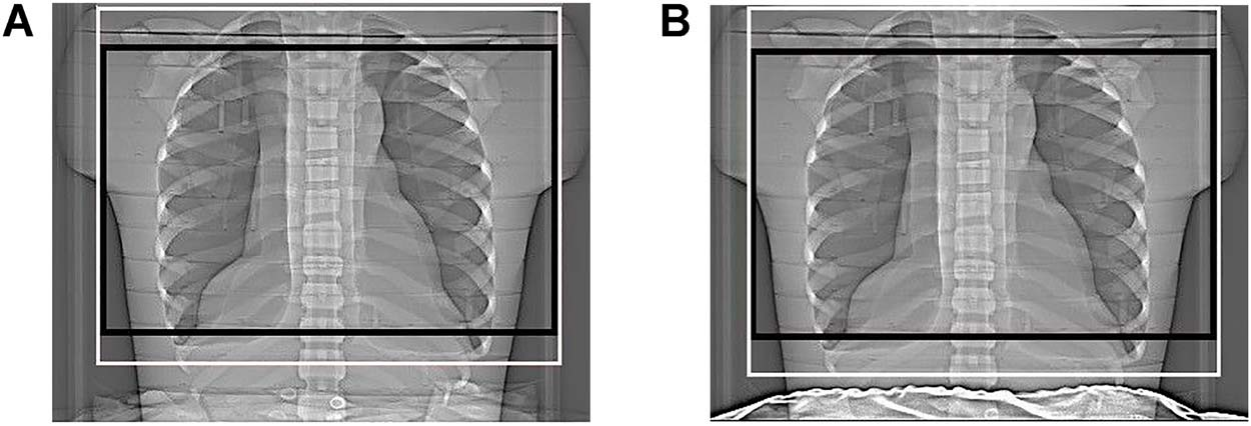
Localiser radiographs showing the CT scan area for standard and short CTPA without shielding (A) and with shielding (B). The white box corresponds to the long scanning range; the black box corresponds to the short scanning range.
